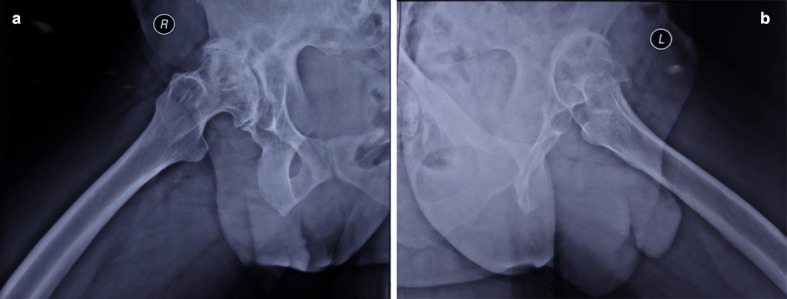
a) Right hip X-ray lateral view, b) Left hip X-ray lateral view.
